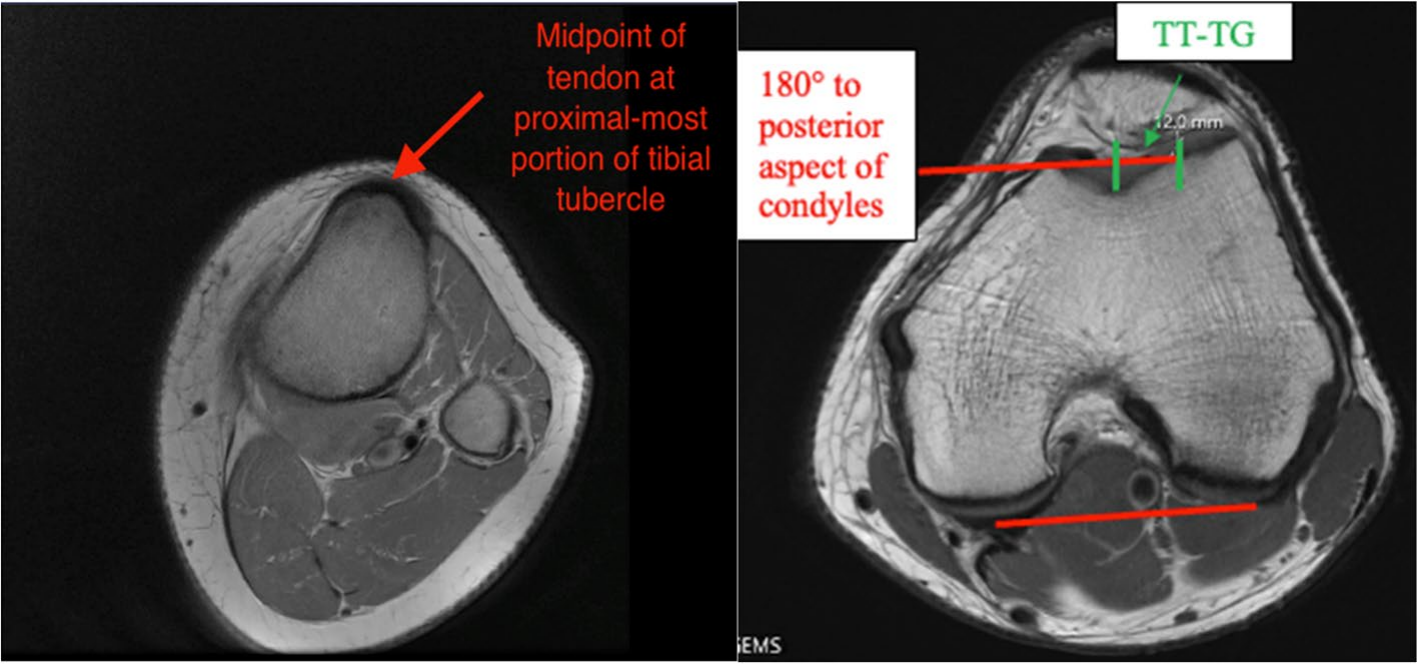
Tibial tubercle-trochlear groove distance measurement on axial MRI
